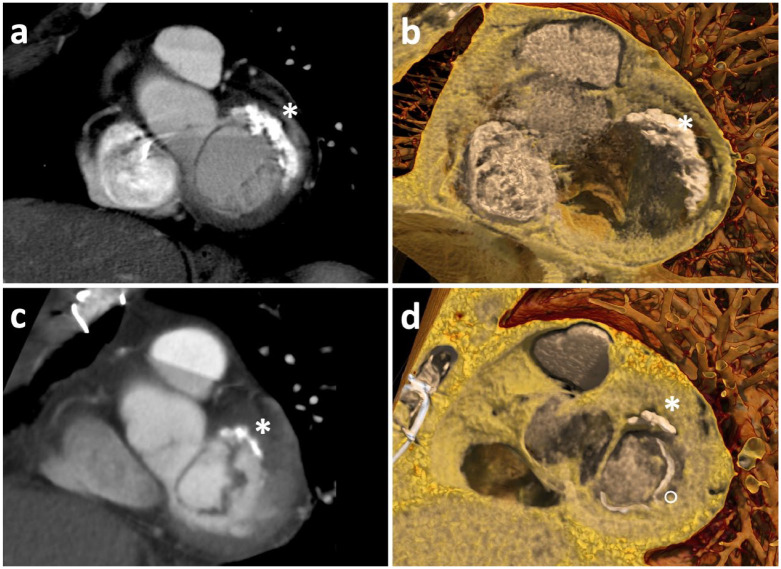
(a) Preoperative CT of the heart demonstrating severe MAC (*). (b) Global illumination
